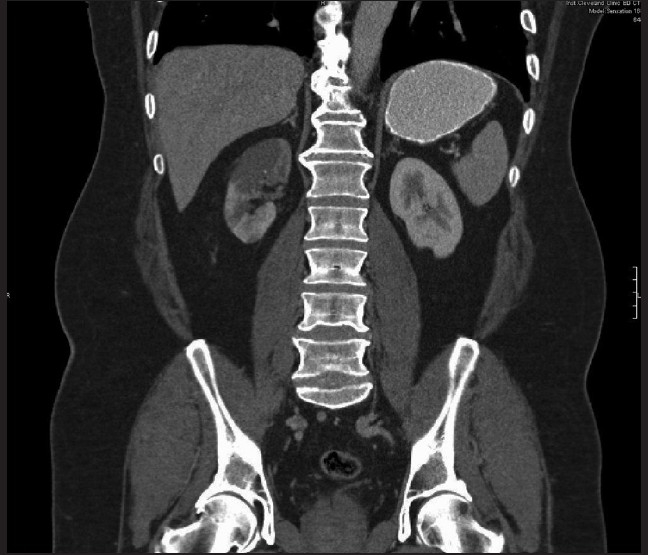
Axial computed tomography enhanced with IV contrast exhibiting minimal enhancement of the right kidney with some spared areas of the periphery, consistent with a cortical rim sign (spared peripheral circulation via the capsular collateral arterioles)
